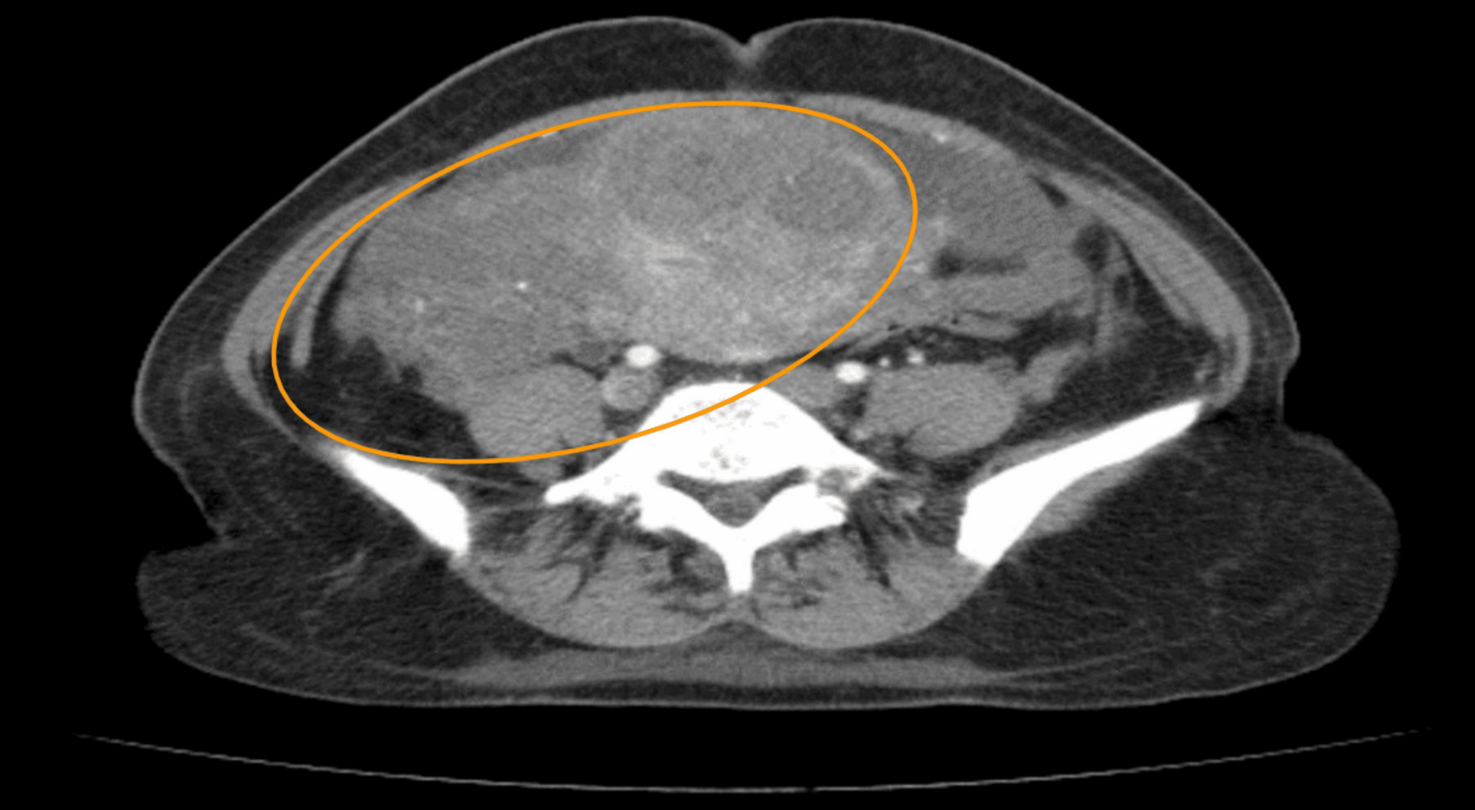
Axial section of contrast-enhanced CT (CECT) in the arterial phase showed a large, lobulated, heterogeneously enhancing soft tissue mass in the pelvis The uterus was not visualized separately from this lesion (indicated by the circle), suggesting either origin from or complete involvement by the mass.
